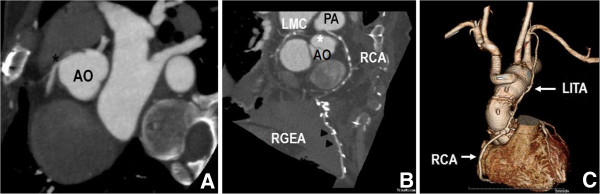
Postoperative images. (A) Post-operative enhanced chest CT scan shows neo-ostium (black asterisk) from the right sinus of Valsalva in patient 1. (B) Post-operative contrast-enhanced CT scan shows the anomalous RCA shared the same sinus with the left coronary system (white asterisk), with its attenuated proximal portion passing between the aorta and pulmonary trunk in patient 2. This image also shows that RGEA was bypassed to the distal RCA. (C) Post-operative three-dimensional volume rendering of contrast-enhanced CT scan in patient 3. Chest CT scan revealed that the ascending aorta and part of the aortic arch were replaced with a prosthetic graft, and that the innominate artery was bypassed using a side-arm branch. Also the left internal thoracic artery to the proximal right coronary artery bypass was patent. (CT: computed tomography; AO: aorta; LMC: left main coronary artery; PA: pulmonary artery; RCA: right coronary artery; LITA: right internal thoracic artery; RGEA: right gastroepiploic artery).
